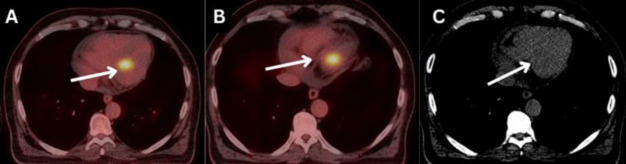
^18^F-FDG PET/CT scan, axial planes of fused images (A & B) showing an intense focus of FDG activity (SUV_max_:10.8) within the left ventricle (white arrows) measuring about 0.4×0.3 cm in maximal active component which correlate to a soft tissue density mass on corresponding CT component (C) (white arrow)
